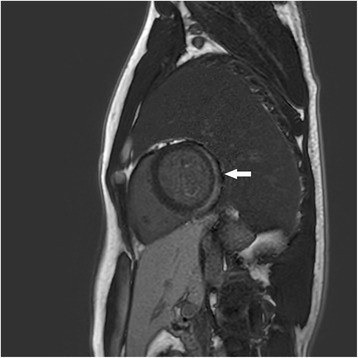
MRI imaging in the short axis. Posterior and posterolateral late gadolinium enhancement in the subepicardial region in with acute myopericarditis (Patient 1)
